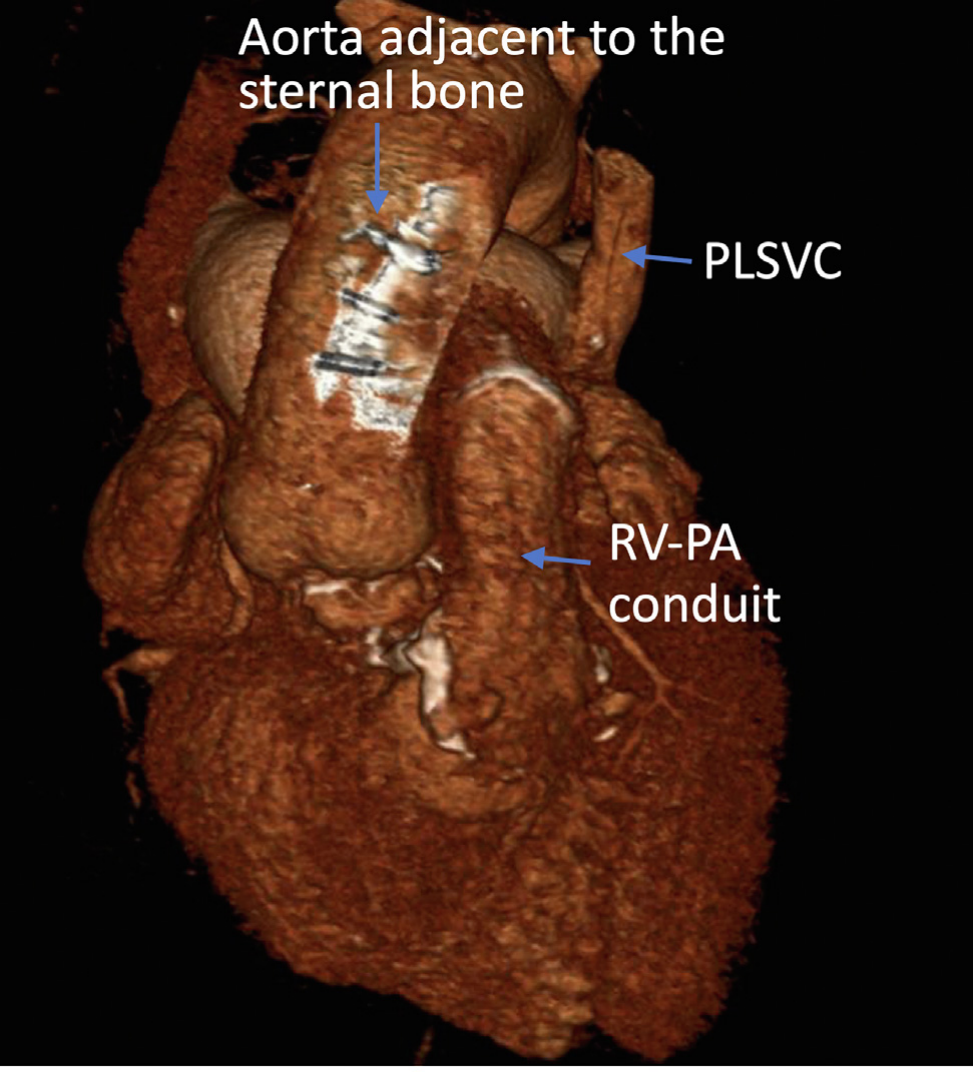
Three-dimensional reconstruction of computed tomography scan. The aorta was adjacent to the sternal bone. *PLSVC*, Persistent left superior vena cava; *RV-PA*, right ventricle-pulmonary artery.
